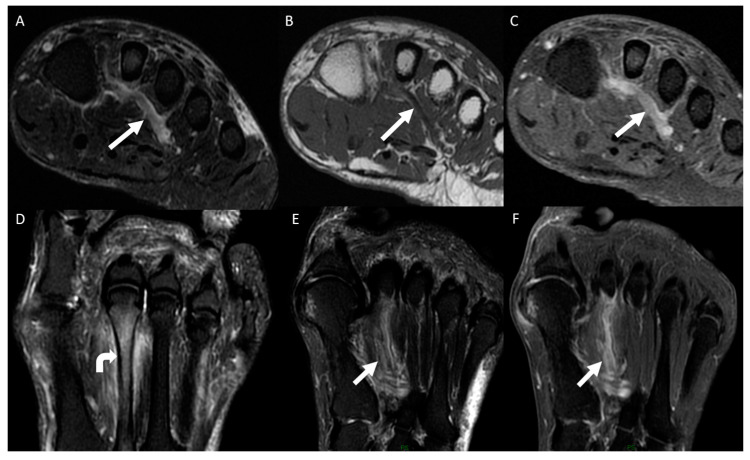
A 64-year-old woman presented with left foot plantar pain experienced while walking, persisting for 5 days. Forefoot MRI images in the short-axis plane, including a T2-weighted fat-suppressed scan (A) and a T1-weighted scan (B), as well as a post-gadolinium T1-weighted fat-suppressed scan (C), and in the long-axis plane, a T2-weighted fat-suppressed scan (D,E), along with a post-gadolinium T1-weighted fat-suppressed scan (F), revealed thrombosis in the metatarsal vein (arrows). This condition was characterized by venous dilation, with intraluminal thrombus and an intravenous filling defect. Image (D) displays a stress fracture of the second metatarsal (curved arrow), along with bone marrow edema and adjacent soft tissue edema.
